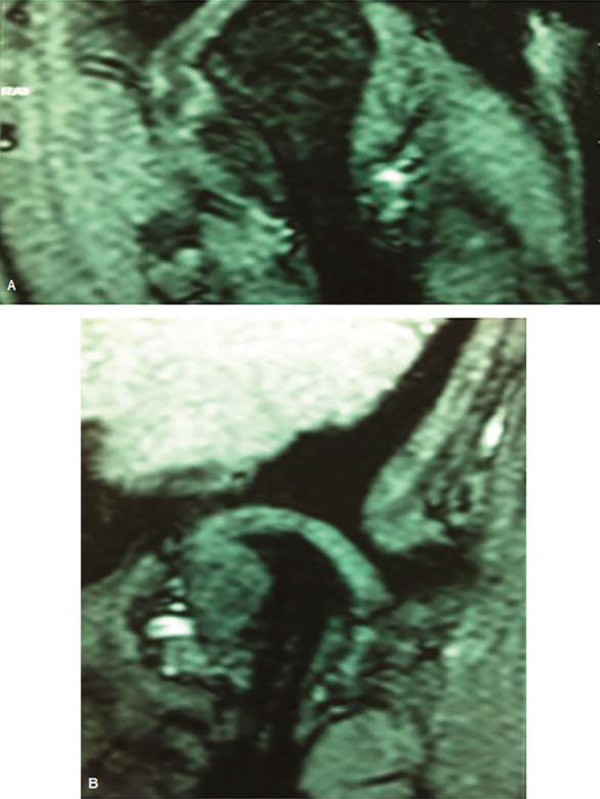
Magnetic resonance image evidencing integrity of condyle cortical on the right side (A) and a high degree of condylar resorption on the left side (B) of a patient presenting posterior open bite on the unaffected side
